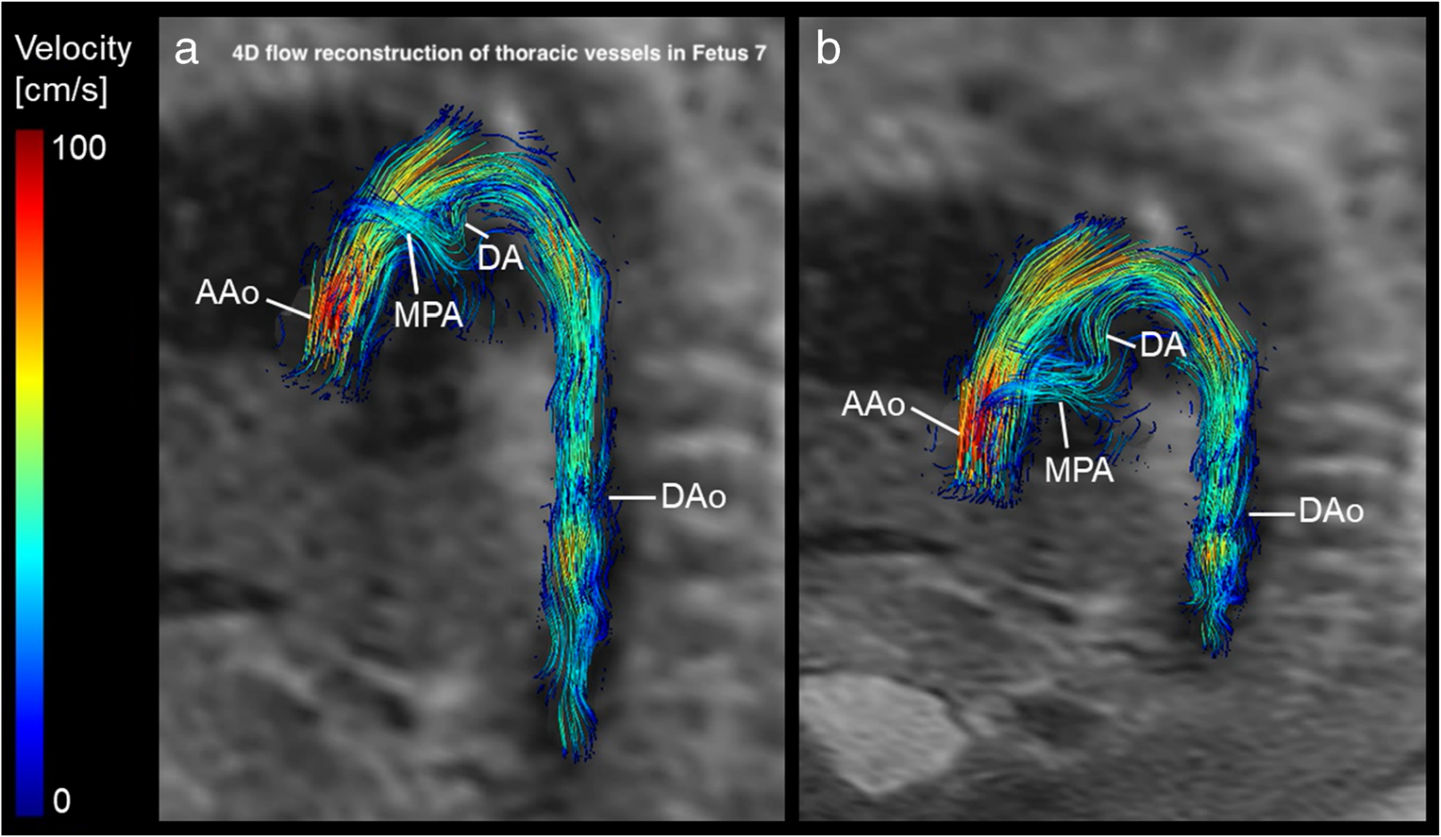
4D flow MRI–based reconstruction with T2-weighted image overlay (systolic phase) in sagittal (a) and left cranio-lateral view (b) of the fetal great thoracic vessels with known echocardiographic diagnosis of right-sided aortic arch (gestational age 33^+3^ weeks). 4D flow MRI–based reconstruction with various angulation of the thoracic vessel anatomy showed right-sided aortic arch (in combination with transversal T2-weighted imaging) and ductus arteriosus (DA) originating from the main pulmonary artery (MPA) (b). DUS-gated 4D flow MRI indicated no double aortic arch anomaly. AAo, ascending aorta; DAo, descending aorta
